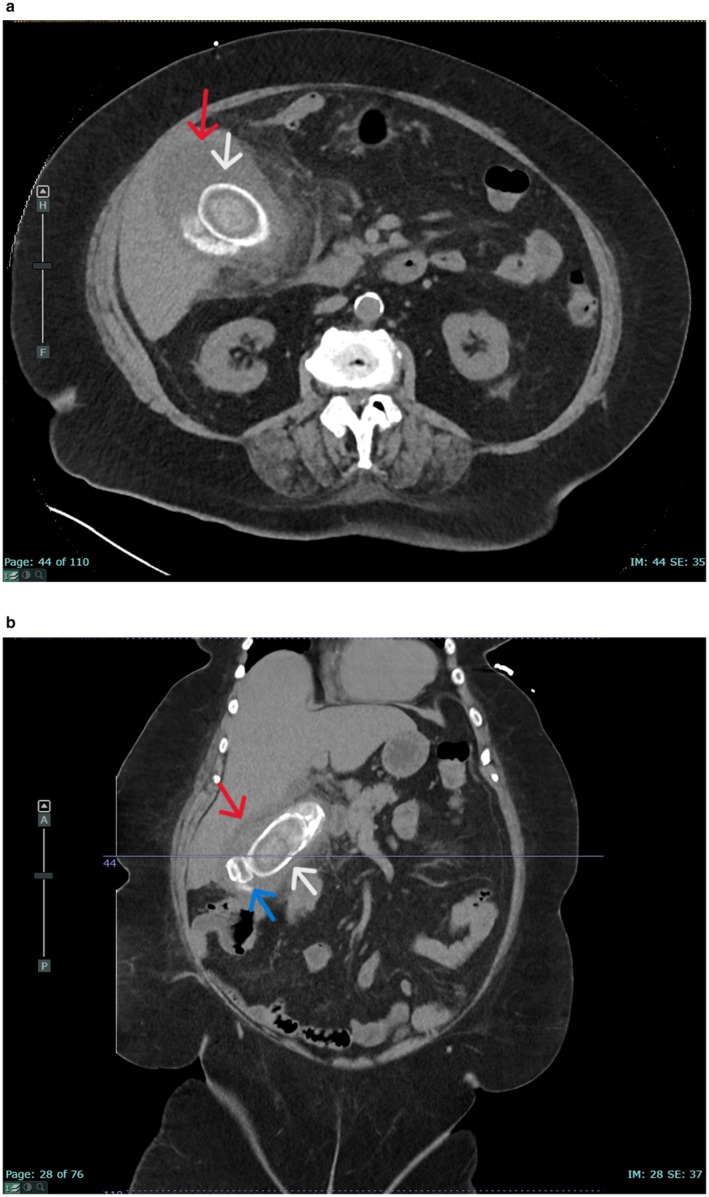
Initial computed tomography scan of the abdomen demonstrating large, calcified gallstones in (a) axial and (b) coronal views. Arrows marking calcified gallstone initially reported as porcelain gallbladder given large size and ovoid shape (white) and secondary gallstone (blue) in non‐calcified gallbladder (red).
